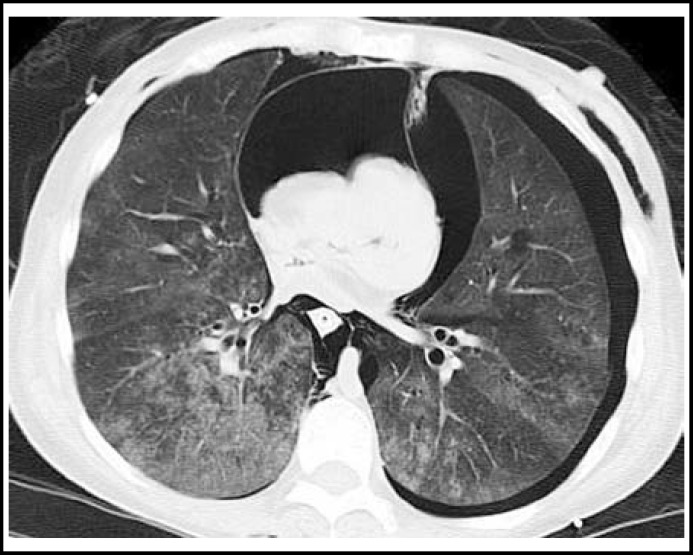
CT showing subcutaneous emphysema, bilateral pneumothorax, tension pneumopericardium and compressed heart
